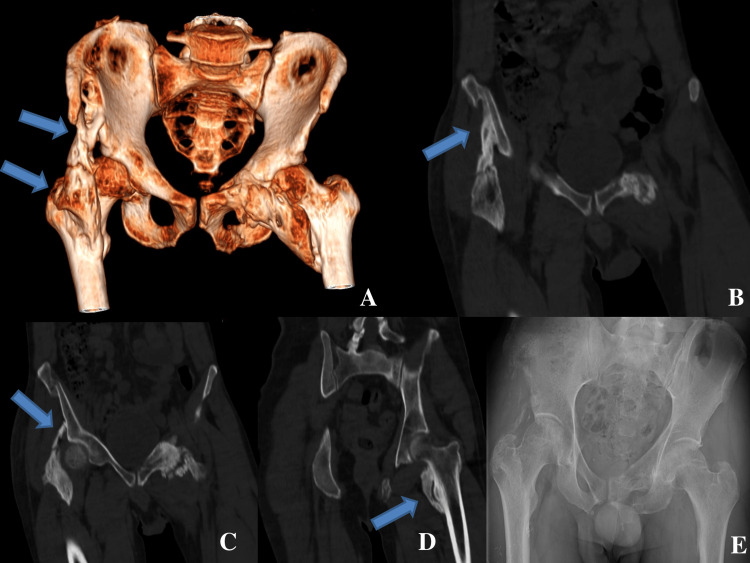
Pre- and postoperative imaging of the second patient A: preoperative pelvis 3D-scan image; B: right hip osteoma: proximal iliac implantation; C: right hip osteoma: distal iliac implantation, left hip osteoma: proximal implantation on the iliopubic branch; D: left hip osteoma: femoral implantation; E: pelvis X-ray at the final follow-up
